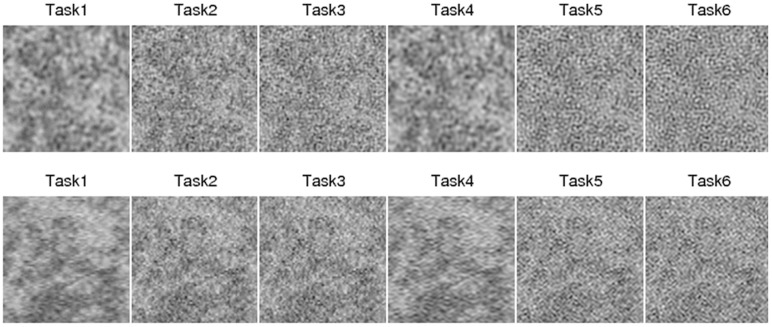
Reconstructed CBCT images. Reconstructed CBCT images for the transverse (upper) and the longitudinal plane (lower). The display window was [0.10, 0.39] *cm*^−1^.
